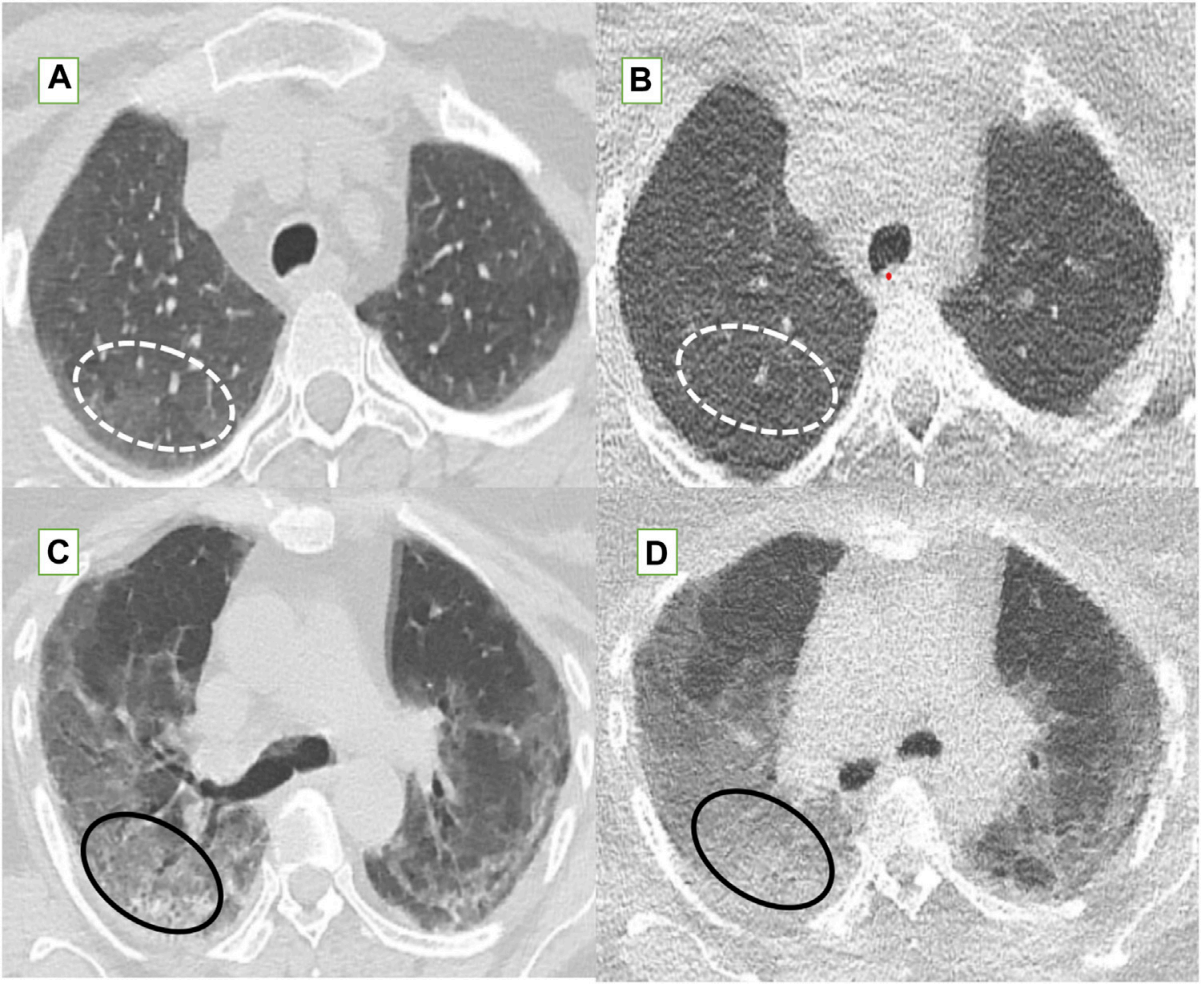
The fallacies of ultra-low dose CT (ULDCT) chest (right column) compared to standard dose CT (SDCT) chest (left column) have been depicted. (A,B) In a 46-year-old class 2 obese patient, SDCT (A) showed GGOs (dotted white elliptical circle) which were completely missed on the corresponding ULDCT images (B). (C,D) In another 53-year-old class 3 obese patient, the SDCT chest (C) showed GGOs (black elliptical circle) which were interpreted as consolidation on the corresponding ULDCT images (D).
